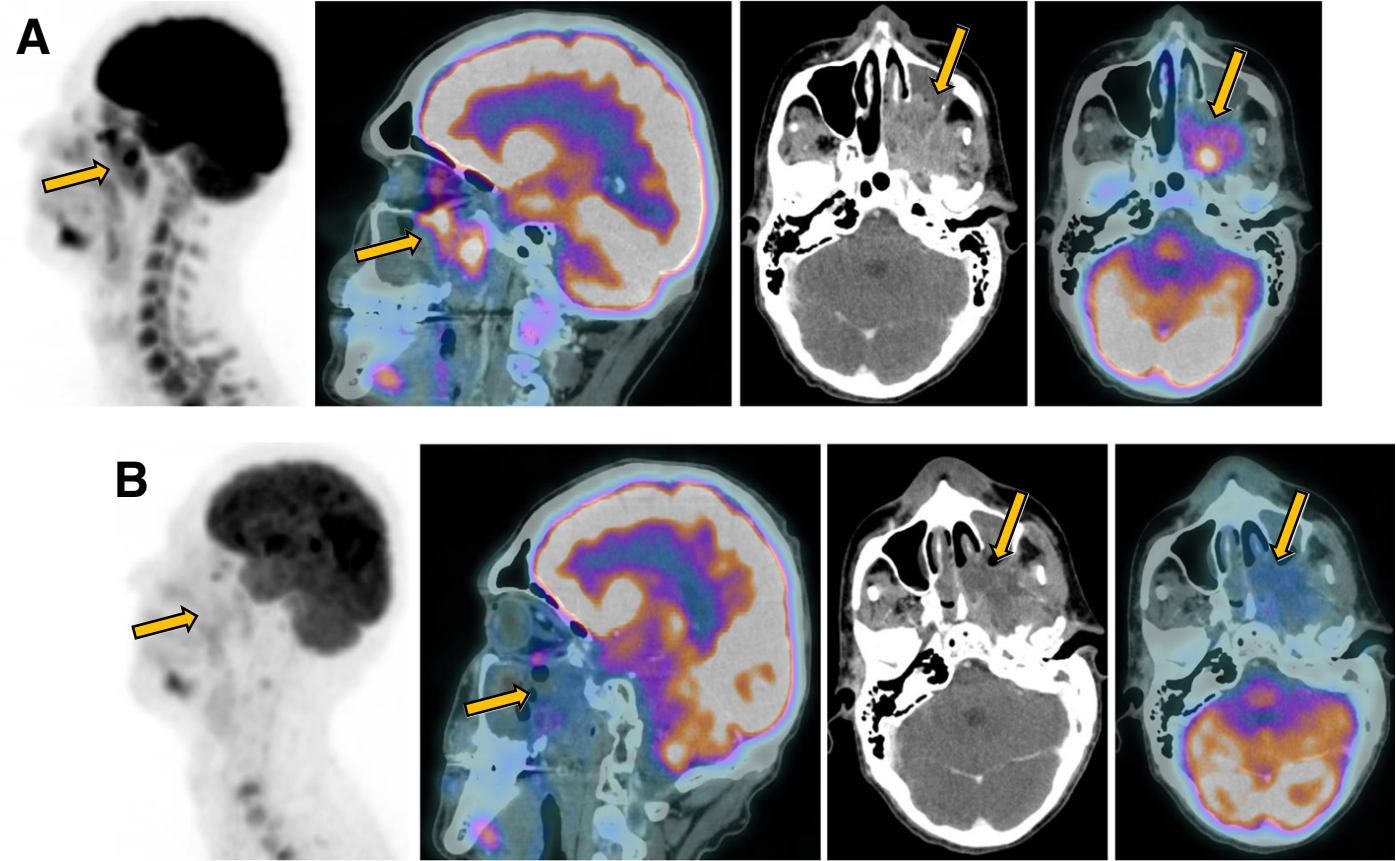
Local complete metabolic response seen in ^18^F-fluorodeoxyglucose positron emission tomography-computed tomography. Fluorodeoxyglucose-positron emission tomography-computed tomography head and neck images from baseline before second-line chemotherapy (a) showing locally advanced left maxillary sinus enhanced mass (*arrows*) with fluorodeoxyglucose avidity. Positron emission tomography-computed tomography of 1 month after end of treatment (b) confirming morphological shrinkage of the left maxillary sinus with extensive necrosis (*arrows*) without any fluorodeoxyglucose uptake, representing local complete metabolic response
